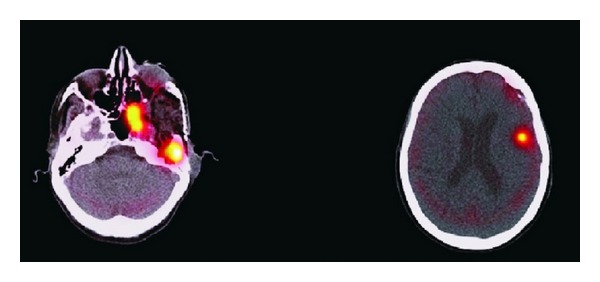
Fused SPECT/CT images of somatostatin receptor scintigraphy demonstrating increased tracer uptake in a patient with anaplastic meningioma [67].
